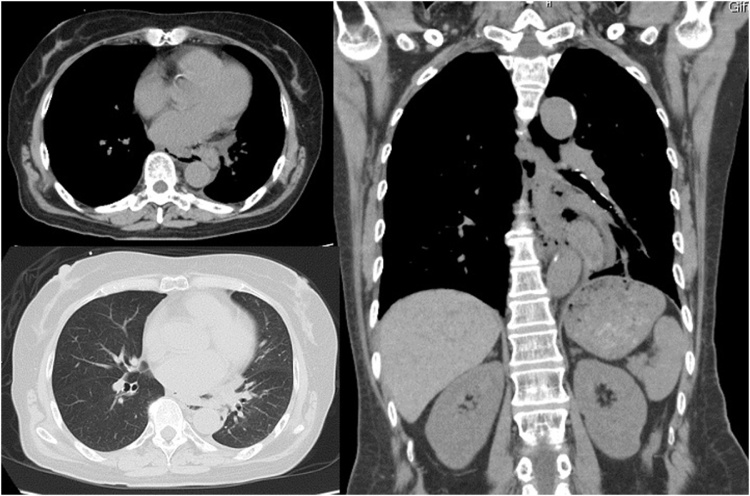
A computed tomography scan showing mediastinal emphysema.
